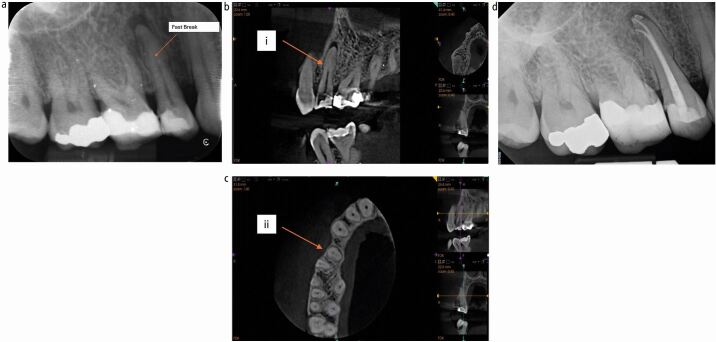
a) Probable canal bifurcation in the plane of the radiograph demonstrating a ‘fast break'. b, c) CBCT of tooth: fast break (i), canal bifurcation evident at mid-root level (ii). d) Completed RCT showing (obturated) bifurcated canal
